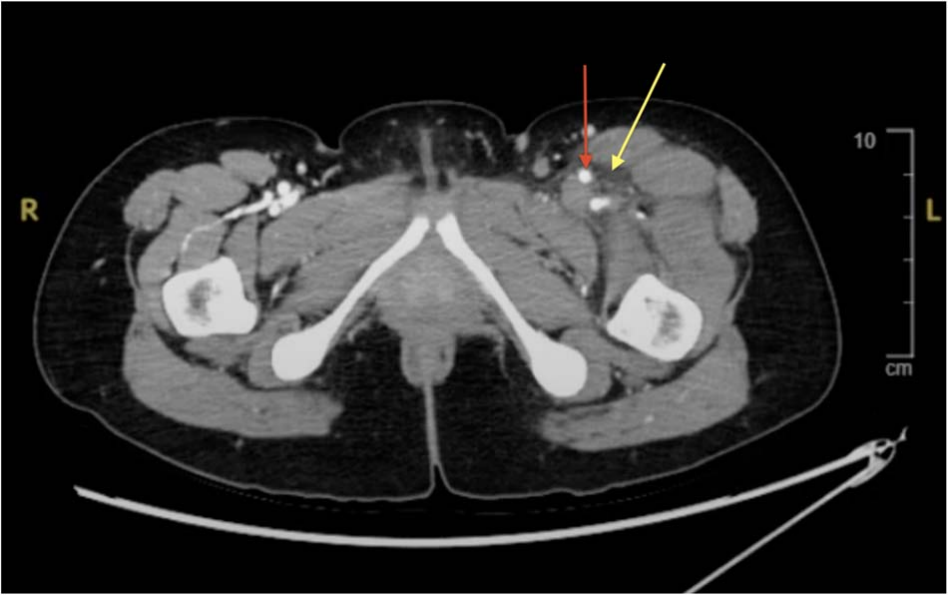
CT angiogram without contrast of the left lower extremity indicating thrombosis of the left common iliac vein with perivascular fat stranding. DVT appears as a non-enhancing filling defect (arrow) within the lumen of the LCIV. Surrounding tissue contains perivascular fat stranding which appear as hazy opacities (arrow). The left lower extremity appears larger than the right due to thrombotic obstruction to venous outflow.
